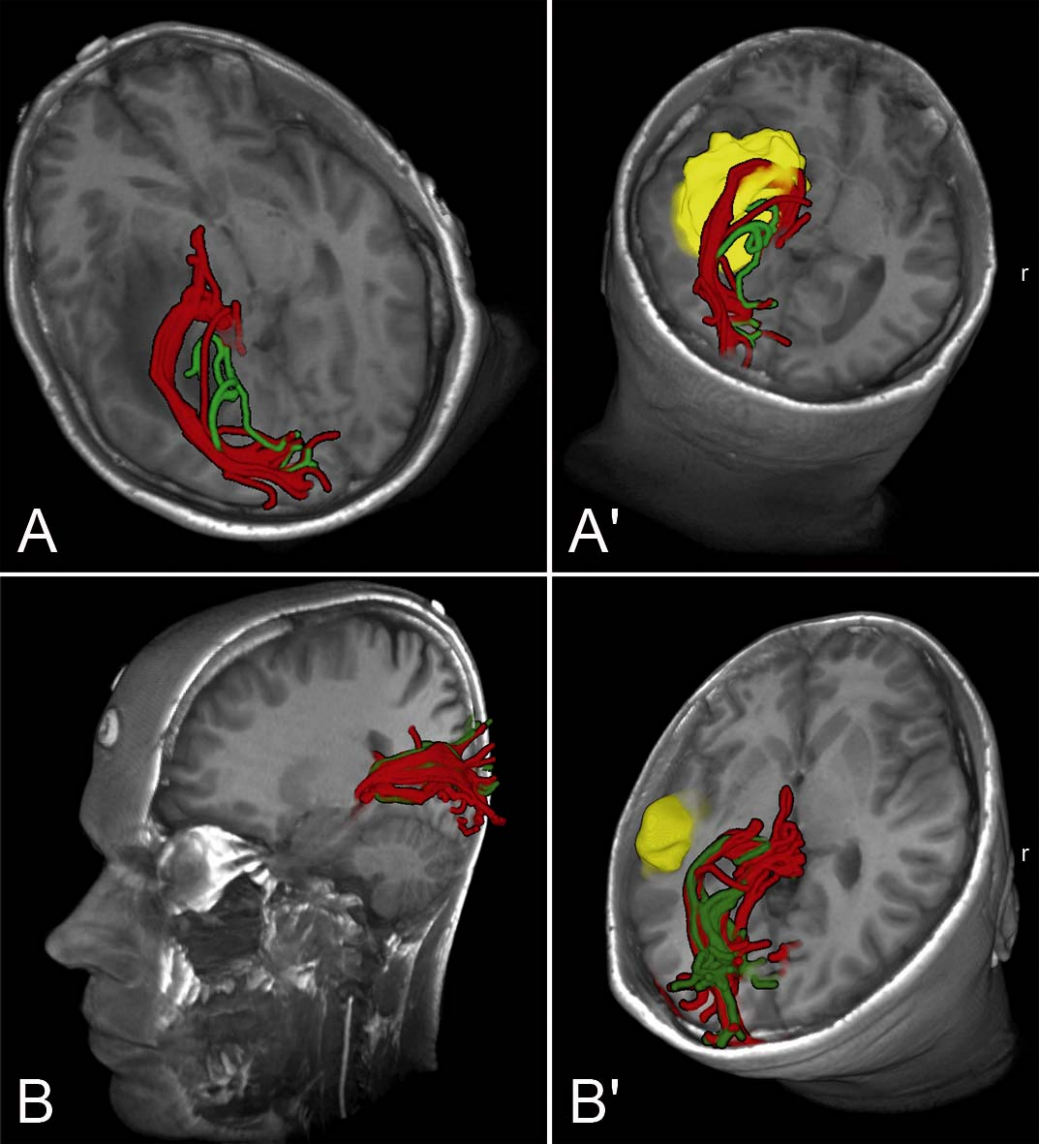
3-D models of T1-weighted MR-images with overlay of HARDI+CS-(red), and DTI-(green)-based tractography of the optic radiation presented as hulled fiber objects. A+A′: Case presentation (case 7): Case of a large temporo-mesial GBM of a 45 year-old male patient, showing the marked differences of HARDI+CS-, and DTI-based fiber objects in case of a large, high-grade tumor. A: axial oblique view. A′: Axial oblique view. Tumor manually segmented in yellow. B+B′: Case presentation (case 6): 35 year-old male patient with small diffuse astrocytoma. Less remarkable differences of tractography results in case of this smaller, low-grade tumor. B: Left sagittal oblique view. B′: Axial oblique view. Tumor manually segmented in yellow.
